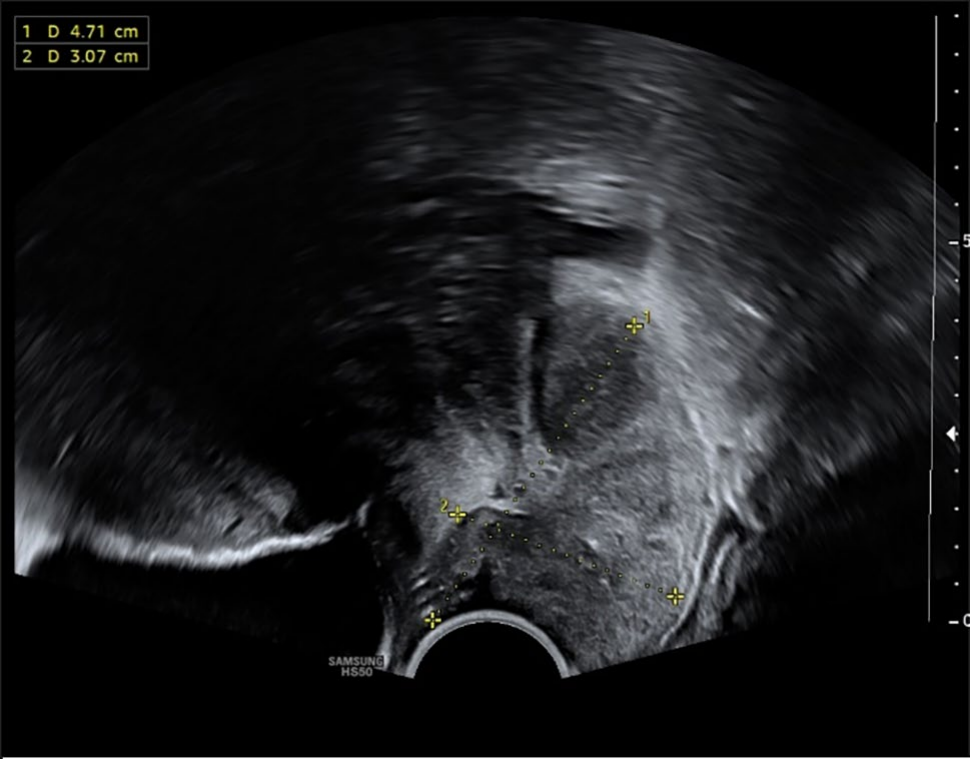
Transvaginal sonogram shows an exophytic tumor of the uterine cervix
